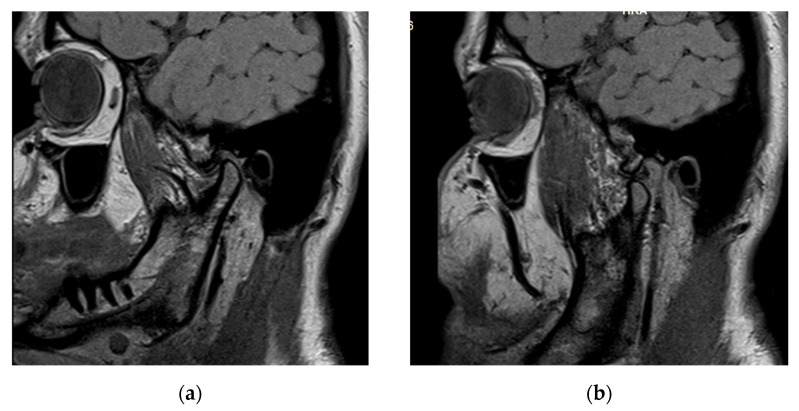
Sagittal (a) closed and (b) open mouth proton density MRI of the right joint: anterior disk displacement with reduction (DDR).
